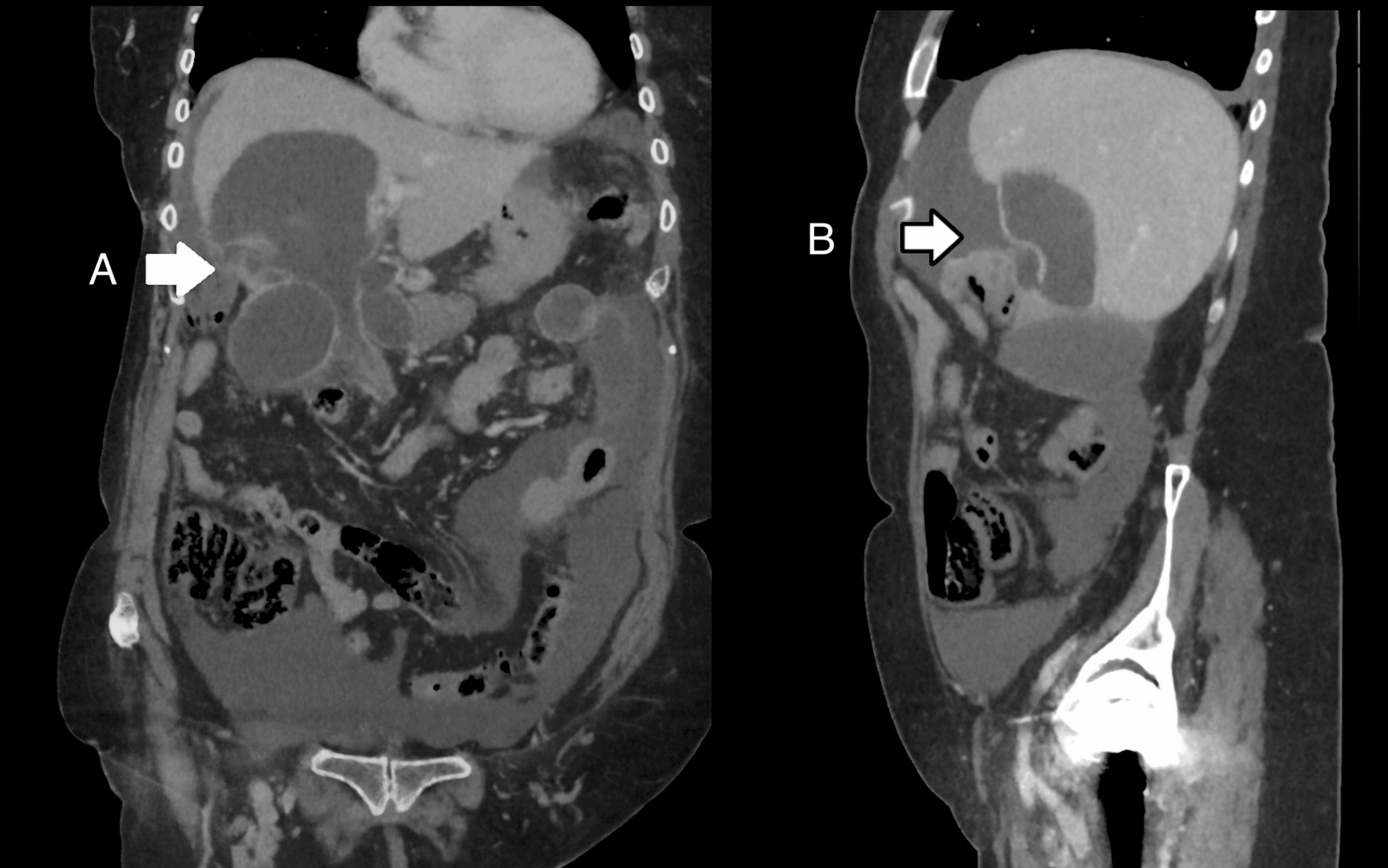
CT Imaging (A) Coronal CT image showing a ruptured hepatic cyst (white arrow) with surrounding low-attenuation fluid tracking along the peritoneal cavity, consistent with cyst leakage.
